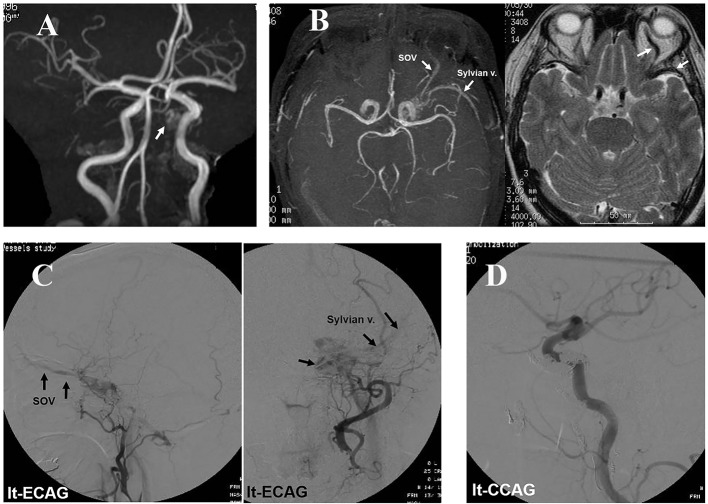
Case 1: (A) MRA obtained 3 months after the initial headache showing an abnormal intensity in the left CS (arrow), but no dilatation of the SOV. (B) MRI demonstrating blood reflux to the left CS, SOV, and Sylvian vein after the patient had become aware of eye symptoms at the eighth month. (C) Angiography showing CS-dAVF reflux to the left SOV and Sylvian vein. (D) Post-embolization angiography showing no blood reflux to the CS.
